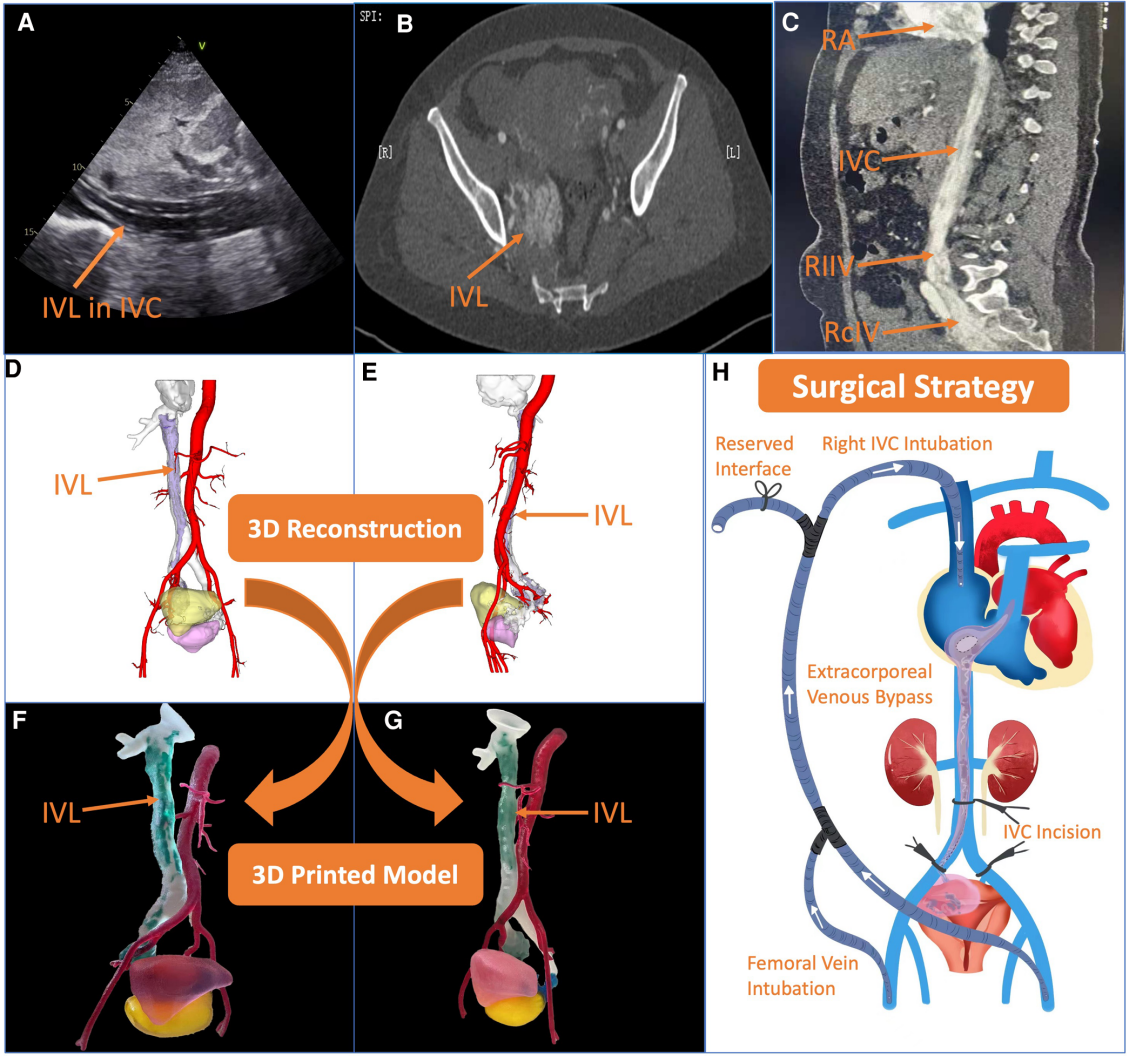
Preoperative assessment and surgical strategy formulation. (A–C) Echocardiography, computed tomography and magnetic resonance imaging showed intravenous leiomyomatosis (IVL) in different anatomical sites clearly. (D,E) 3-dimensional (3D) reconstruction using Materialise 3-matic (Leven, Belgium) software in the lateral view and front view, respectively. The red part is abdominal aorta, the transparent part is inferior vena cava (IVC), the purple part is IVL, the yellow part is bladder, and the fuchsia part is uterus. (F,G) The 3D printed model in the lateral view and front view, respectively. The red part is abdominal aorta, the transparent part is IVC, the green part is cardiac metastatic IVL, the blue part is uterine IVL, the pink part is bladder, and the yellow part is uterus. (H) After comprehensive evaluation, the surgical strategy was decided, transabdominal cardiac metastatic IVL and adnexal hysterectomy were proposed at the same time under off-cardiopulmonary bypass with the establishment of an external venous bypass (bilateral femoral vein-superior vena cava bypass) to maintain circulation.
